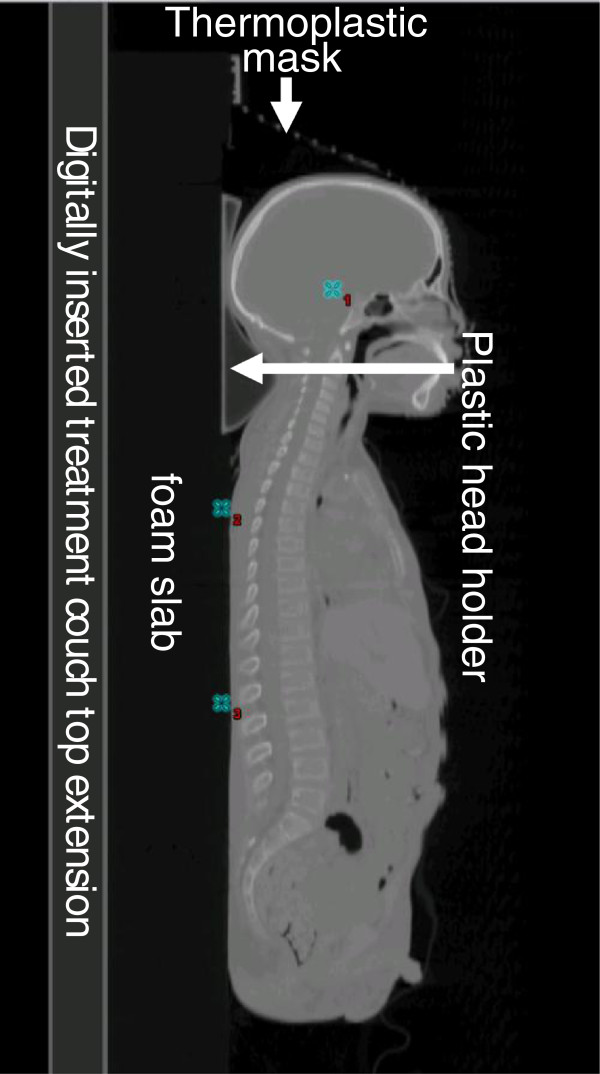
Sagittal CT image showing the patient set-up and immobilization for representative patient (patient 10: male, age 9). The window and level of the image was adjusted to visualize the digitally inserted couch top extension (the portion of the couch that is in contact with the patient), foam pad, thermoplastic mask, and plastic head holder. Isocenters for the cranial field(s), upper spine filed, and lower spine field are indicated with blue × and labeled 1, 2, and 3 respectively.
